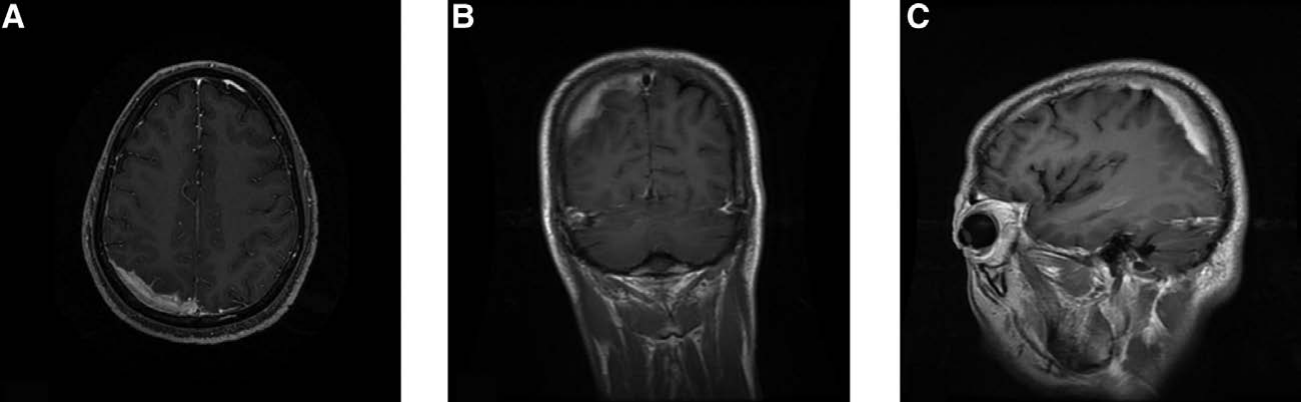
(A–C): Contrast-enhanced axial, coronal and sagittal MR image shows inhomogeneous enhancement of the right parietal lobe mass, involving the sagittal sinus.
